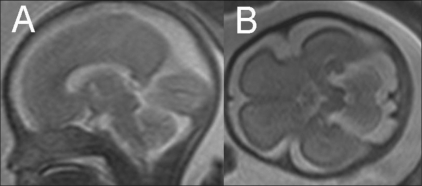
T2W sagittal MRI image (B) at 27 weeks shows the parieto-occipital sulcus (arrowhead), calcarine sulcus (open arrow), and primary cerebellar fissure (closed arrow). A T2W axial MRI image (B) shows myelination (hypointense region) in the posterior brainstem (arrowhead)
